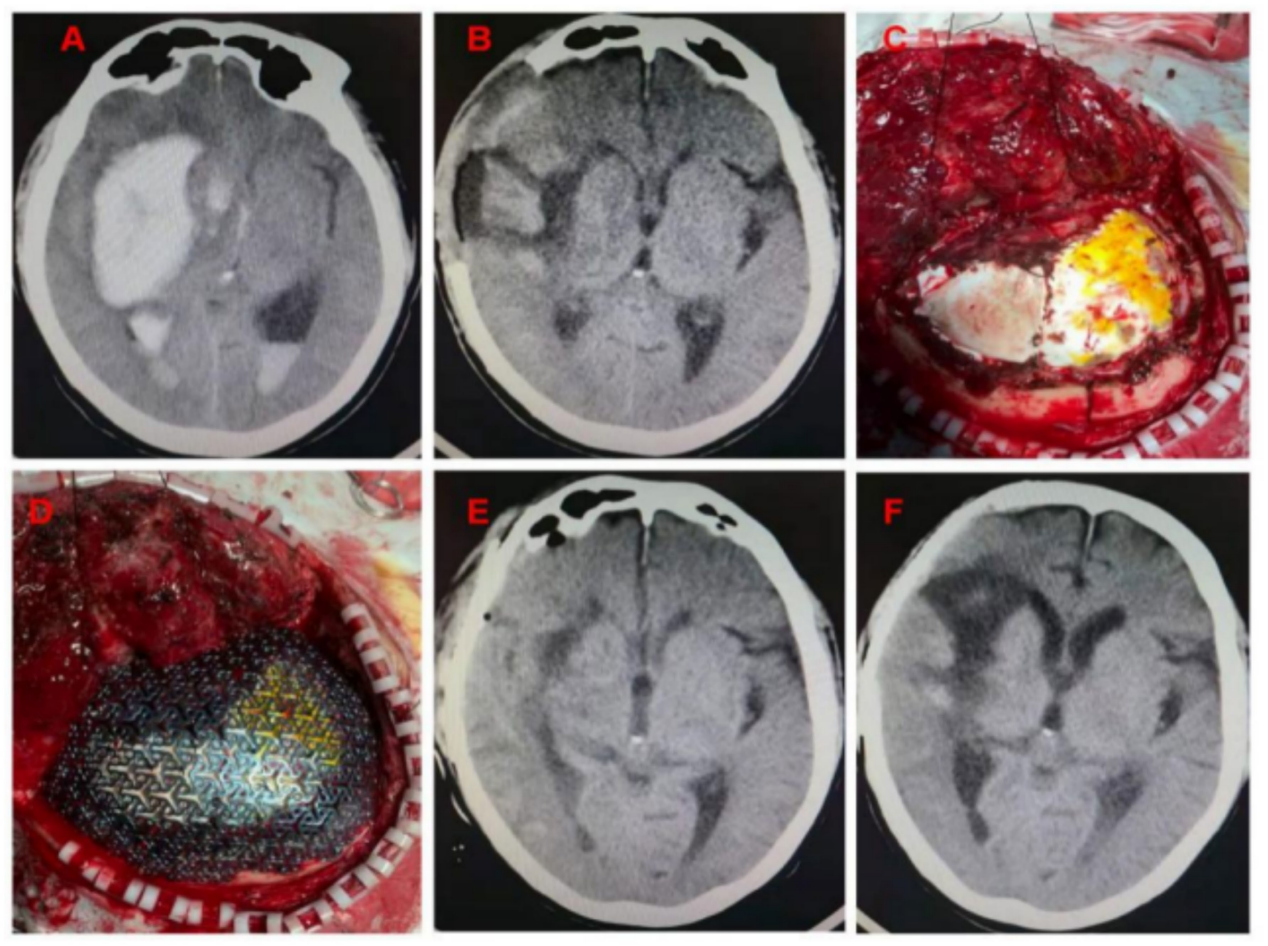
(A) Cerebral hemorrhage in the right basal ganglia region, cerebral hernia, midline displacement approximately 1.6 cm; (B) Seventeen days after DC, the patient’s brain edema decreased, and intracranial pressure was not high; (C) The artificial dura was intact when the flap was turned over. No pseudomembrane was observed. (D) The three-dimensional plastic titanium plate fit well to the skull edge. (E) Head CT examination on the 2nd day after surgery; (F) Head CT was reviewed 1 month after surgery.
